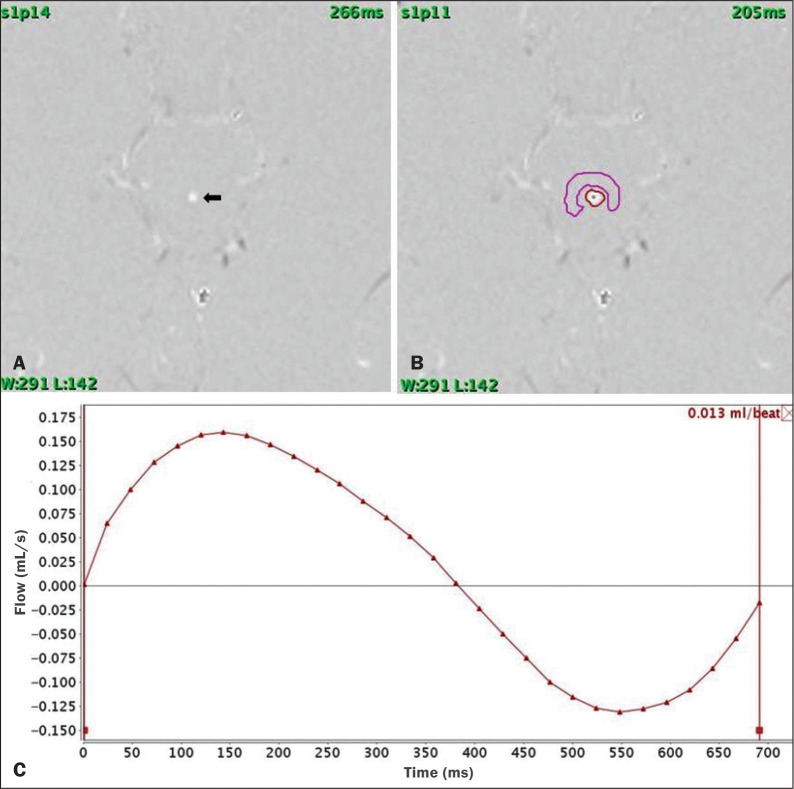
A: Magnified quantitative axial cine-PC MRI sequence at the level of the cerebral aqueduct (arrow). B: Region of interest over the cerebral aqueduct (red). Background subtraction area (fuchsia). C: Flow-time curve obtained during a cardiac cycle.
